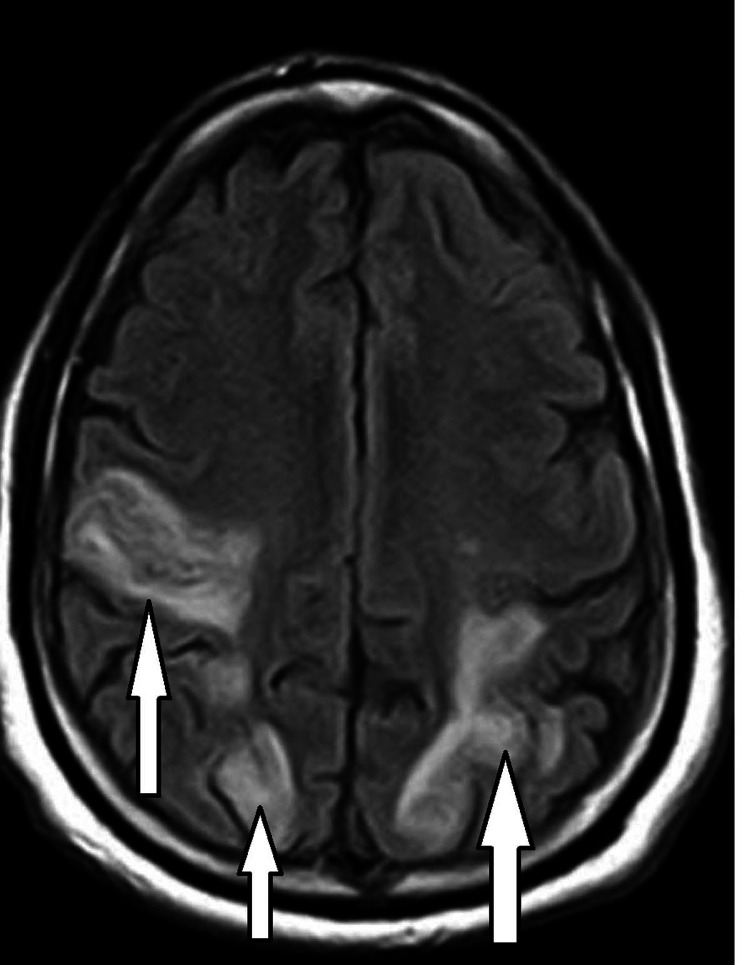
MRI T2/FLAIR image. The arrows signal hyperintensity in the bilateral parietal and occipital lobes. MRI = magnetic resonance imaging; T2/FLAIR = T2-weighted/fluid-attenuated inversion recovery
